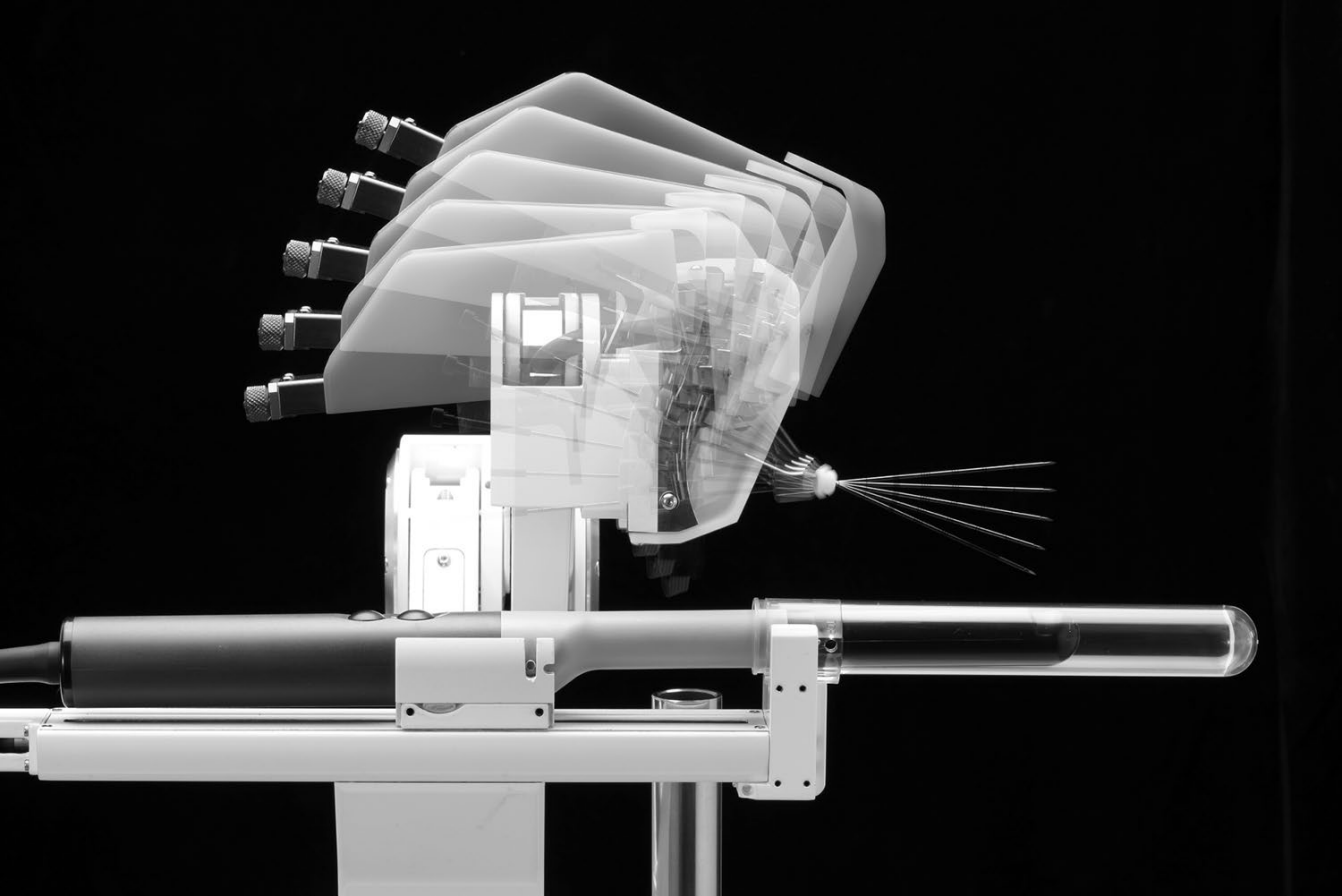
iSR’obot MonaLisa (Biobot Surgical Ltd, Singapore) with BK 3000 transrectal ultrasound probe
